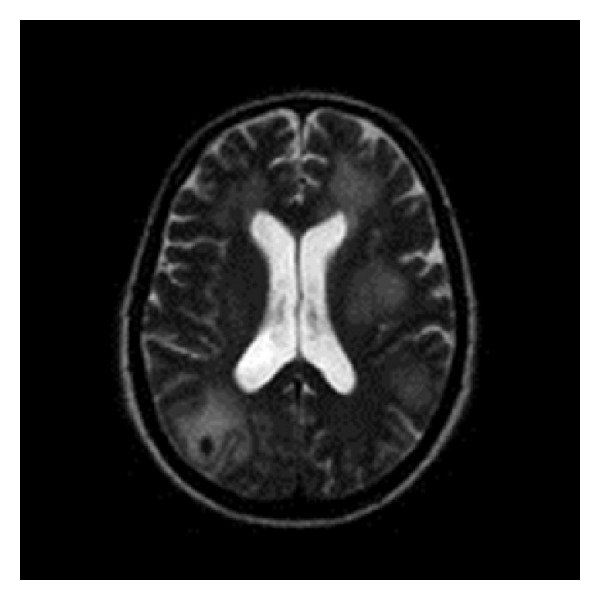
Multiple hemorrhagic foci in bilateral cerebral hemispheres on MRI.
